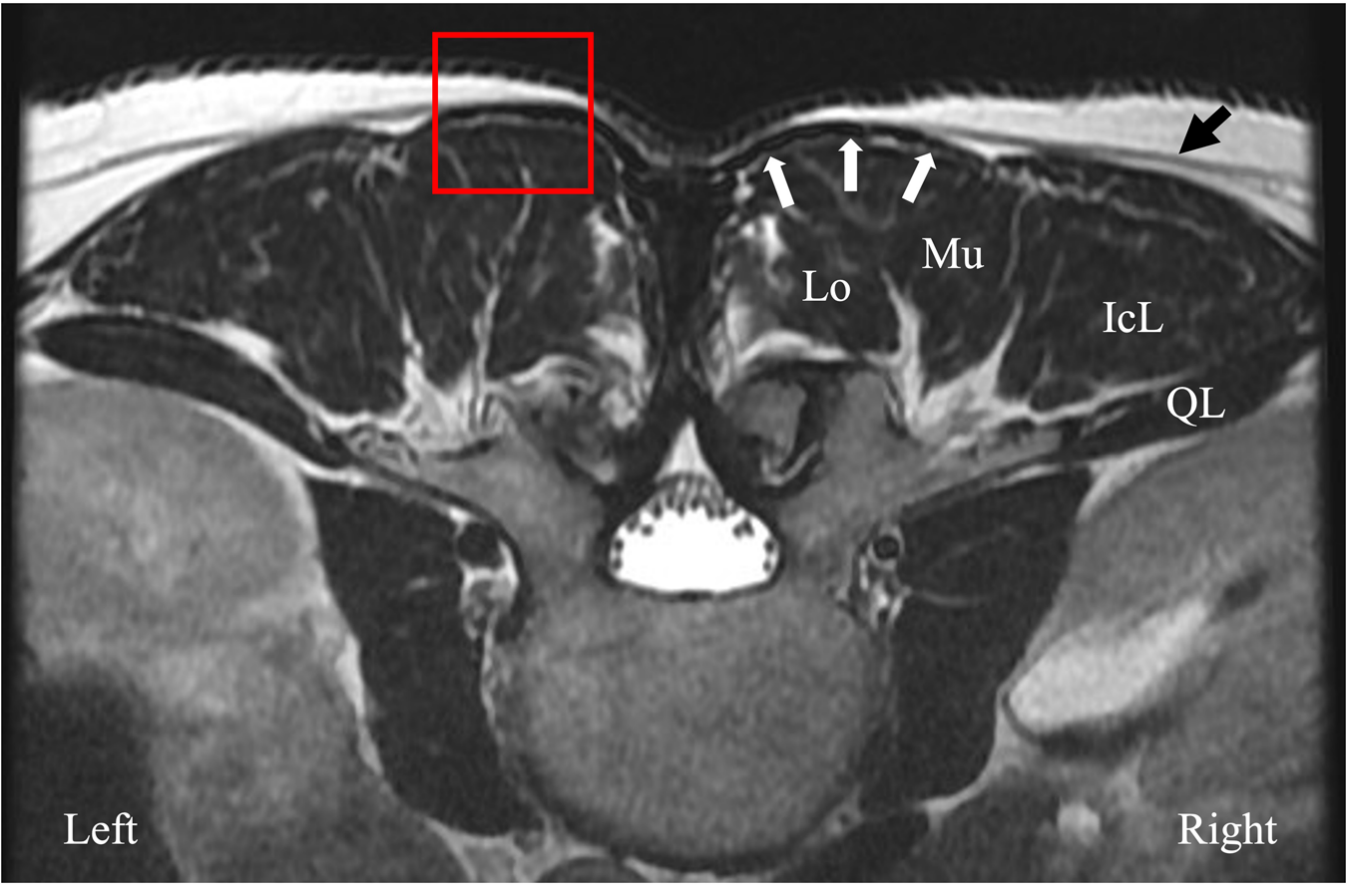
MR imaging of paraspinal soft tissues. Transverse FSE T2 MR image at the L2–L3 interspinous space in a 40-year-old man not involved in the study. The image is displayed to represent a subject in the prone position. The combined aponeuroses of the latissimus dorsi and serratus posterior inferior muscles (not shown here, located laterally) form the superficial lamina of the TLF (black arrow). The deep lamina of the TLF (not shown) is a circular fascia encapsulating the paraspinal muscles. The multifidus and longissimus muscles (ES muscles) are covered by dense ES aponeurosis, highlighted by white arrows. This aponeurosis lies deep in the TLF and attaches to the spinous processes and supraspinous ligament. The red square indicates the region at the peak point of the ES muscles examined by ultrasound. The soft tissue layers included in the square encompass the dermis, subcutaneous adipose tissue, TLF, ES aponeurosis, and muscle depicted on ultrasound in Fig. 3. QL, quadratus lumborum; IcL, iliocostalis lumborum; Mu, multifidus; Lo, longissimus; TLF, thoracolumbar fascia
